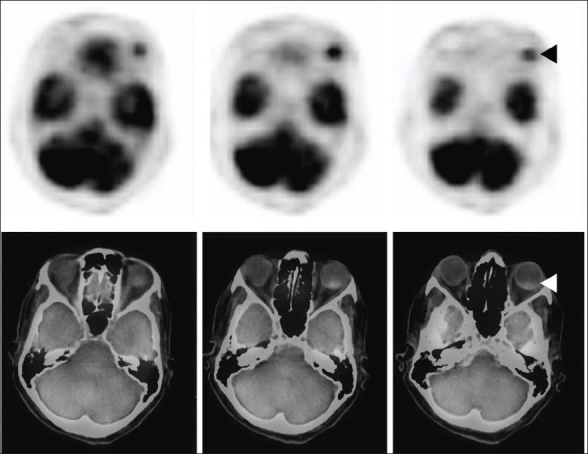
F18 FDG PET CT: Transaxial section across the orbit shows intense focus of FDG activity in the left eye ball posteriorly—fusion image shows coregistration in the posterior choroid corresponding to the radiologic lesion seen in computerized tomogram (◄)
